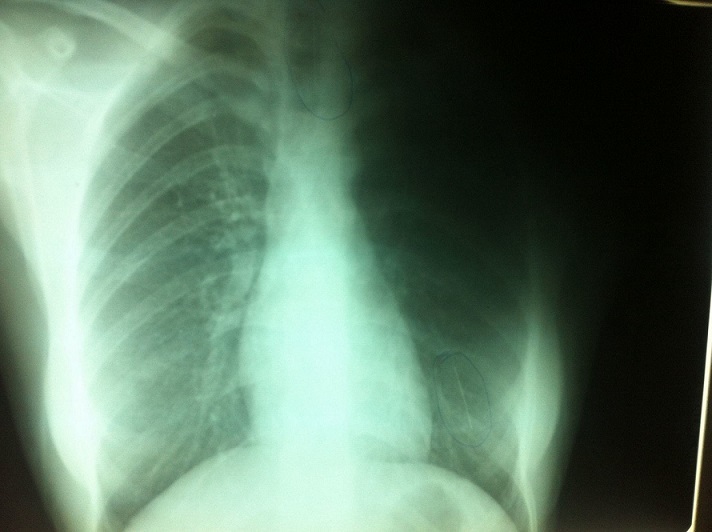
Radiographie thoracique de face montrant deux épingles à foulard: une au niveau de la trachée et l'autre au niveau du segment paracardiaque gauche
